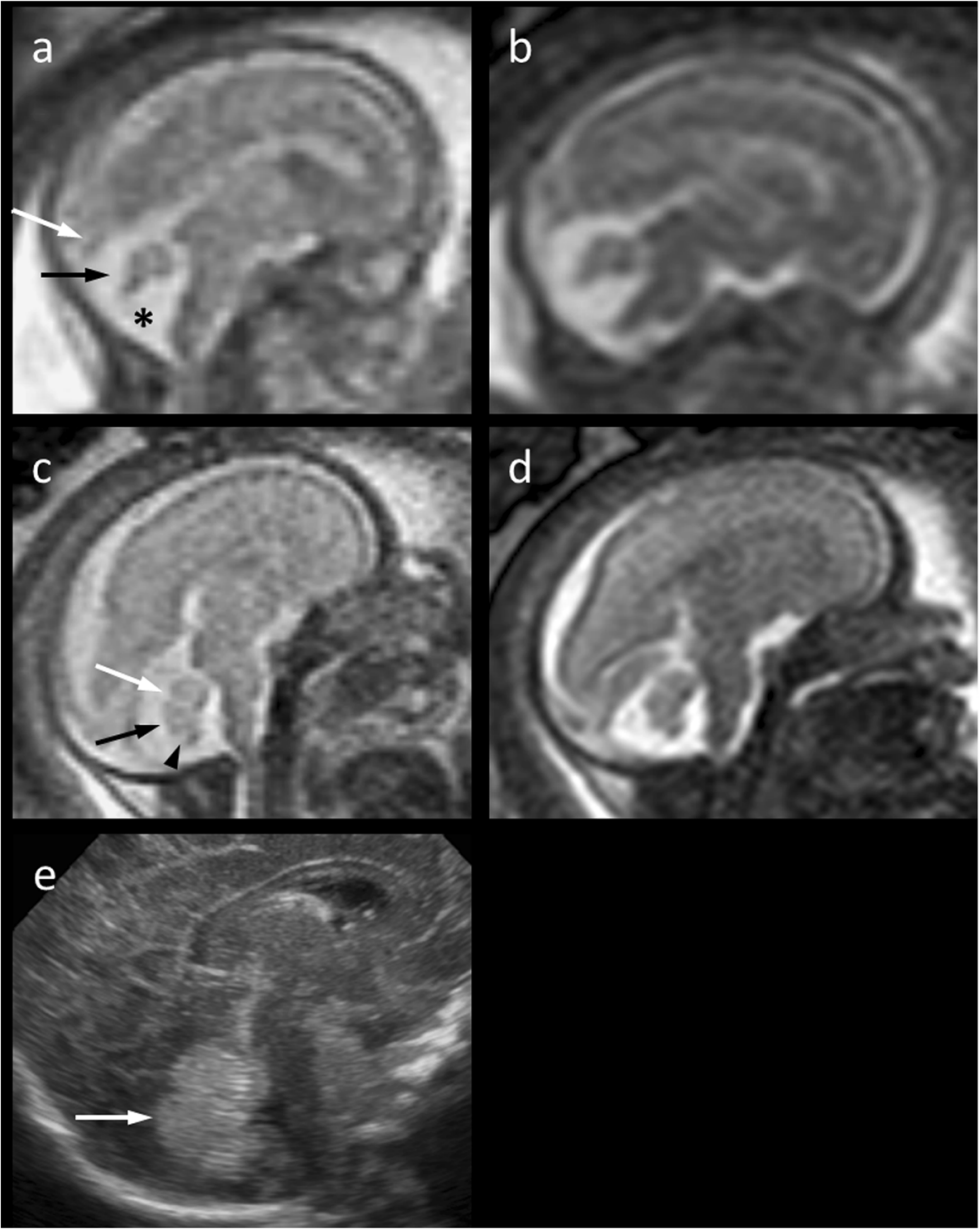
a–d Mid-sagittal T2-weighted 1.5 Tesla magnetic resonance images (a and c, Single-shot; b and d, Balanced Turbo Field Echo) of the fetal brain. At 21 weeks‘gestation (a, b), the cerebellar vermis (a, *black arrow*) appears upwardly rotated and moderately hypoplastic with a normal torcular position (a, *white arrow*). At 27 weeks‘gestation (c, d), the vermis appears nearly normal in position, shape and size suggesting delayed fenestration of Blake’s pouch (a, *asterisk*) The primary (c, *white arrow*), prepyramidal (c, *black arrow*), and secondary (c, *arrow head*) fissures are roughly discernible. e A slight uncertainty regarding minimal hypoplasia of the cerebellar vermis (*arrow*) remains even after inconspicuous transcranial ultrasound postnatally at the age of 10 weeks
